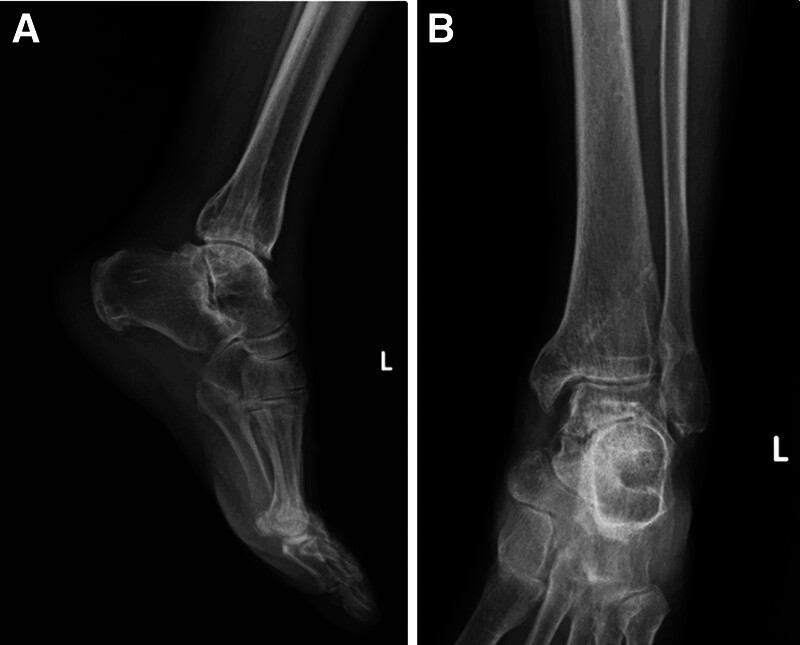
AP (A) and lateral (B) radiographs obtained 5 years after surgery demonstrated acceptable mortise of the left ankle joint.
